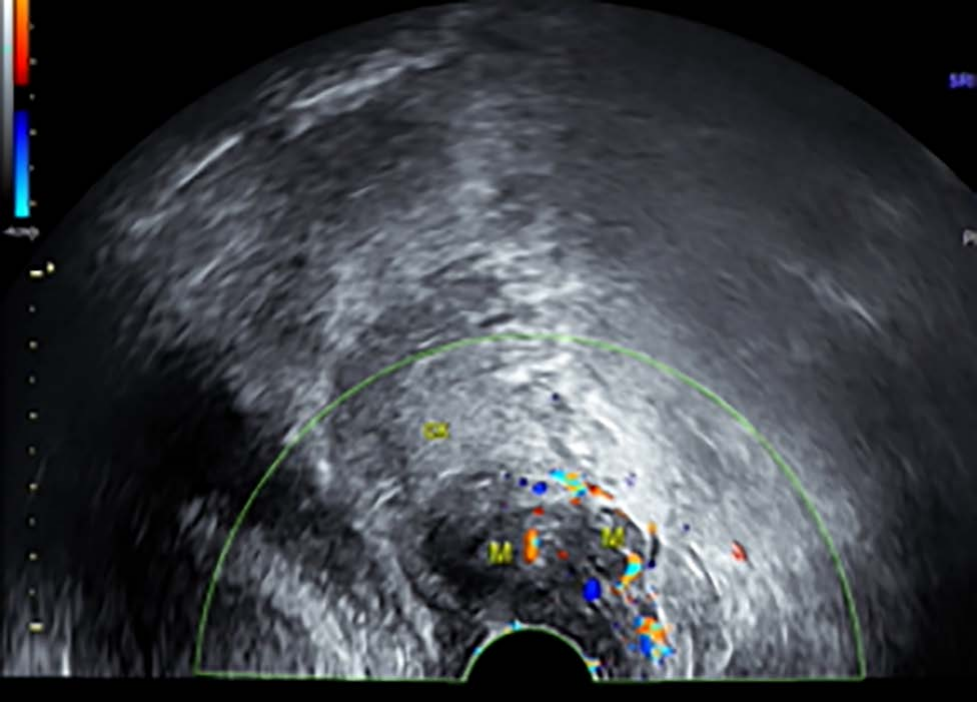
Transvaginal ultrasonography showed low echogenicity in the cervix and low echogenicity in the vagina (excluding cervical fibroids) (a low echogenicity area of approximately 25 × 22 × 20 mm in size and approximately 13 mm in diameter can be seen on the left side of the vagina, closely related to the left anterior wall of the cervix).
